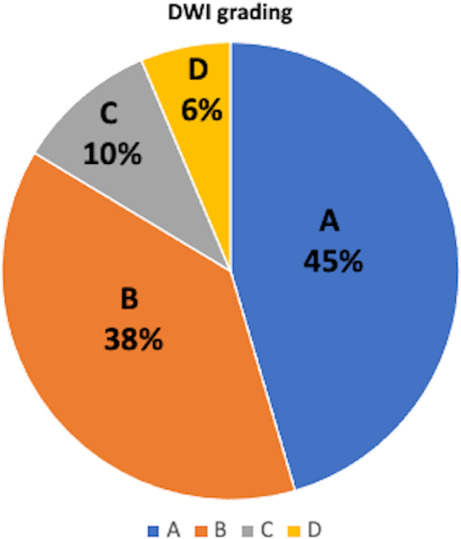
Distribution of DWI grading. Grade A, no high-intensity areas (HIAs); Grade B, small HIAs (≤ 5 spots and each ≤ 10 mm); Grade C, some small HIAs (> 5 spots and each ≤ 10 mm); and Grade D, large HIAs (at least one spot > 10 mm). *DWI* diffusion-weighted imaging, *HIA* high-intensity area
